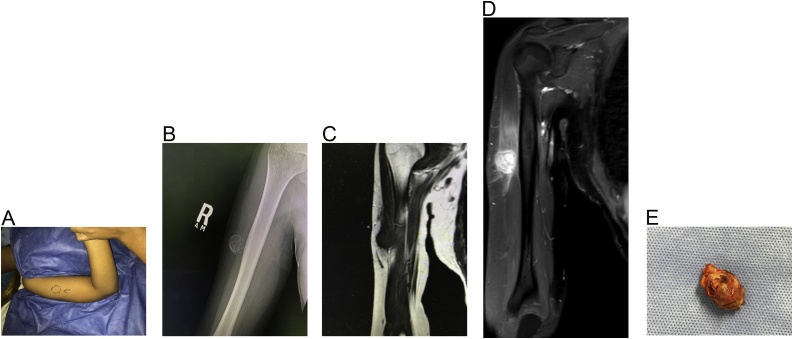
A: the site of the mass in the deltoid muscle. B: plain X-ray showing the calcified mass. C: T1 MRI image showing a hypointense lesion. D: T2 MRI image showing the mass with high intensity and internal heterogenous enhancement. E: The excised calcified mass.
